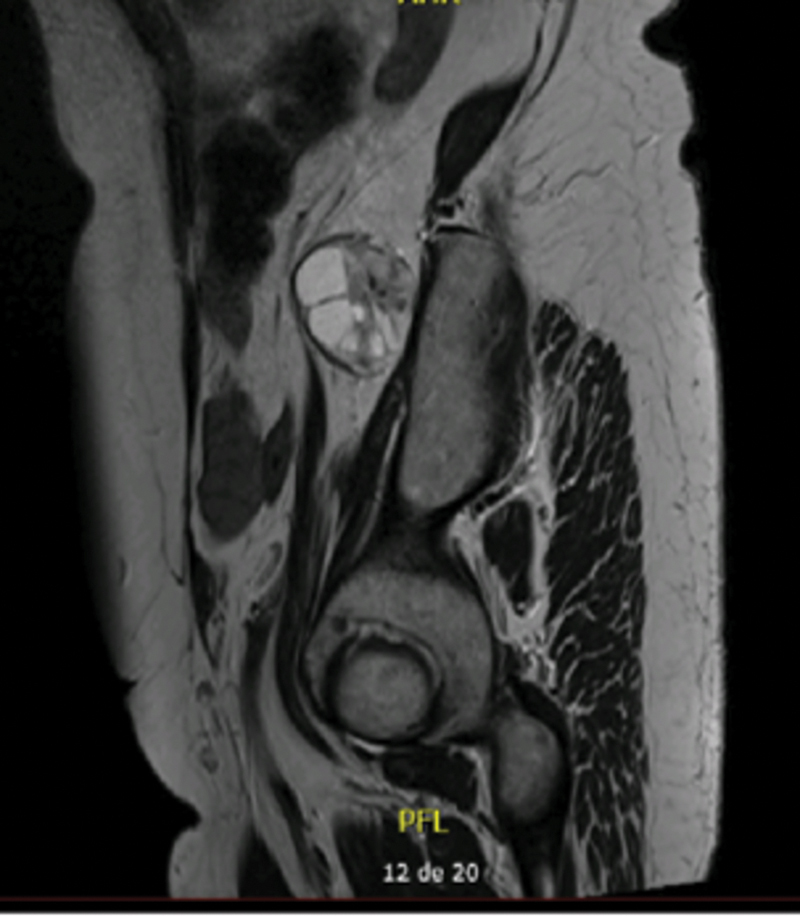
MRI (sagittal plane) – T2- weighted sequence: heterogeneously hyperintense lesion, with few cystic areas, suggestive of neurogenic lumbar plexus tumour.
